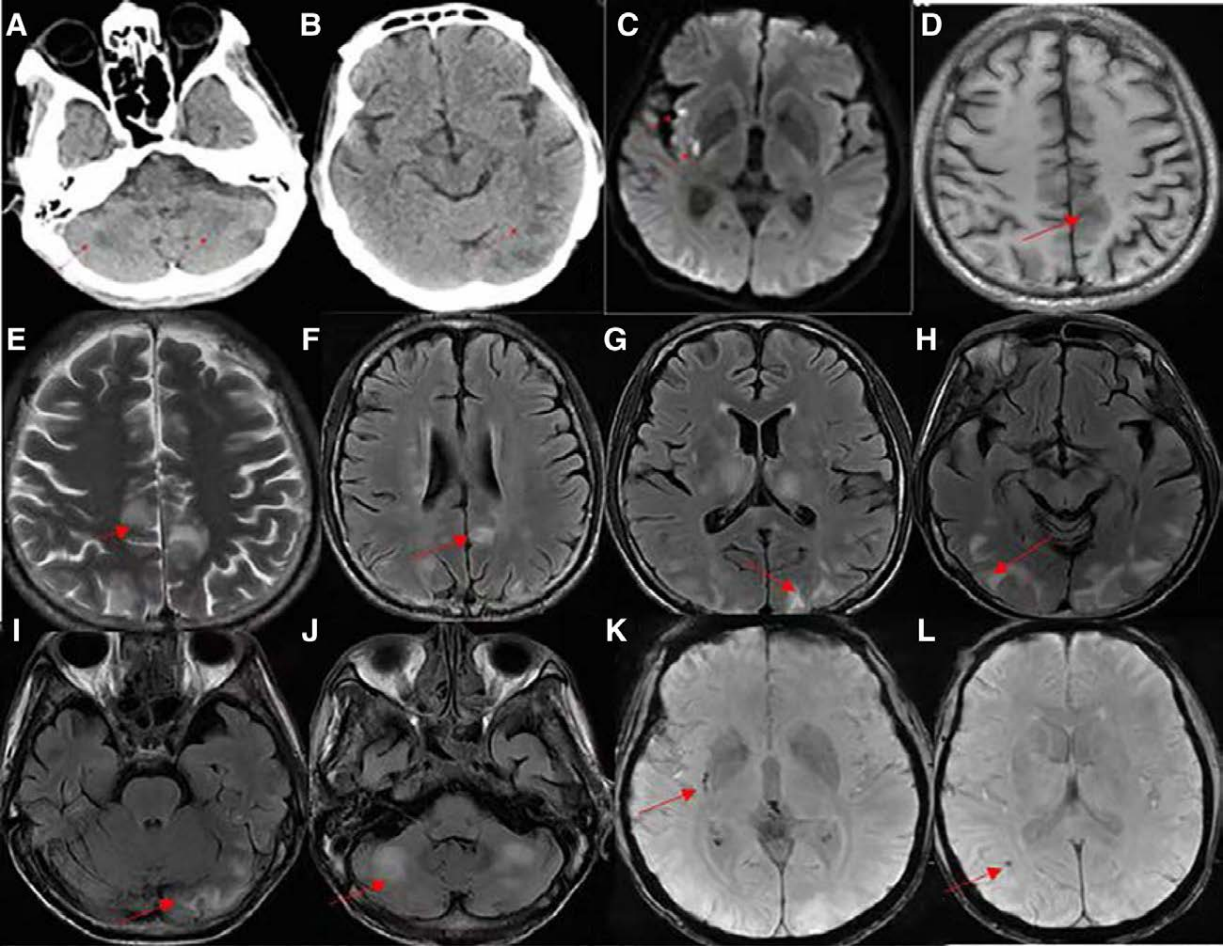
(a, b): CT double frontal-parietal junction cortex, bilateral occipital lobe, bilateral thalamus, and bilateral cerebellar hemisphere lamellar low-density shadow. (c): The right basal ganglia, right insular lobe and abnormal signal shadow of left parietal lobe on DWI?. (d~l): MRI + SWI: d: brain T1W1 symmetrical hypointense shadow in the cerebral hemisphere; (e): symmetrical hyperintense shadow in brain T2W2; (f~j): symmetrical hyperintensities in bilateral basal ganglia, bilateral brain and cerebellar hemisphere of T2Flair brain; (k~l): low signal shadow and micro-hemorrhage in the right basal ganglia area and right temporal occipital junction area of brain SWI. CT = computed tomography, DWI = diffusion-weighted imaging, MRI = magnetic resonance imaging, SWI = sensitive weighted image.
